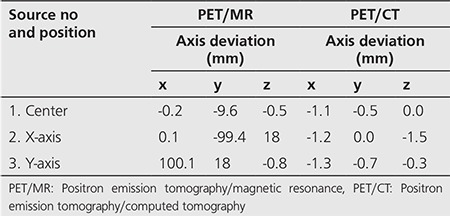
Deviations in the position of the sources for spatial resolution measurement in positron emission tomography/magnetic resonance and positron emission tomography/computed tomography equipment
